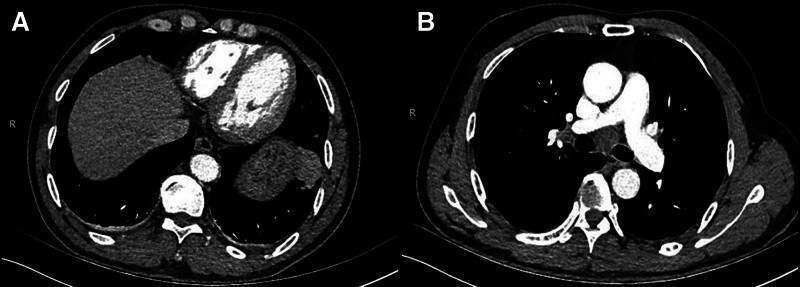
Enhanced chest CT showed that the left lower lobe pulmonary mass was approximately 3.3 cm × 2.3 cm in size, not clearly demarcated from the adjacent diaphragm (A), with slightly lobed edges and contrast-enhanced masses, enlargement of hilar or mediastinal lymph nodes (B).
